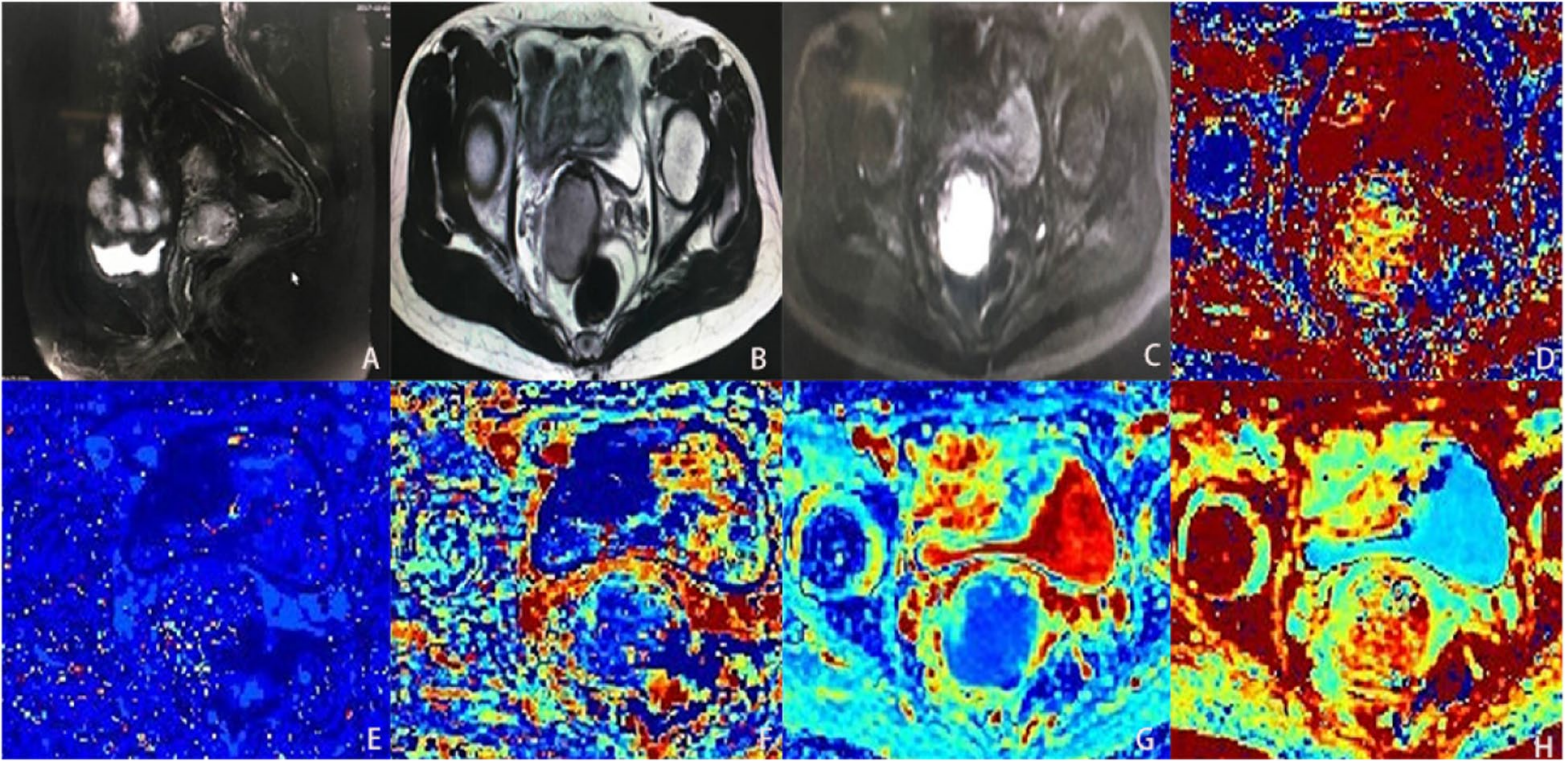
Moderately differentiated squamous cell carcinoma of the cervix. A is sagittal T2WI, and the tumor tissue showed slightly high signal intensity. B is axial T2WI, and the tumor tissue showed slightly high signal intensity. C is DWI (b = 800s / mm2), and the tumor tissue showed slightly high signal intensity. D is the pseudo color image of parameter D in IVIM, and the cancer tissue is yellowish. E is the pseudo color image of parameter D* in IVIM, and the cancer tissue is of partial color. F is the pseudo color image of parameter f in IVM, and the cancer tissue is bluish. G is the pseudo color image of parameter MD in DKI, and the cancer tissue is bluish, and the cancer tissue is bluish. H is the pseudo color image of parameter MK in DKI, and the cancer tissue is reddish
